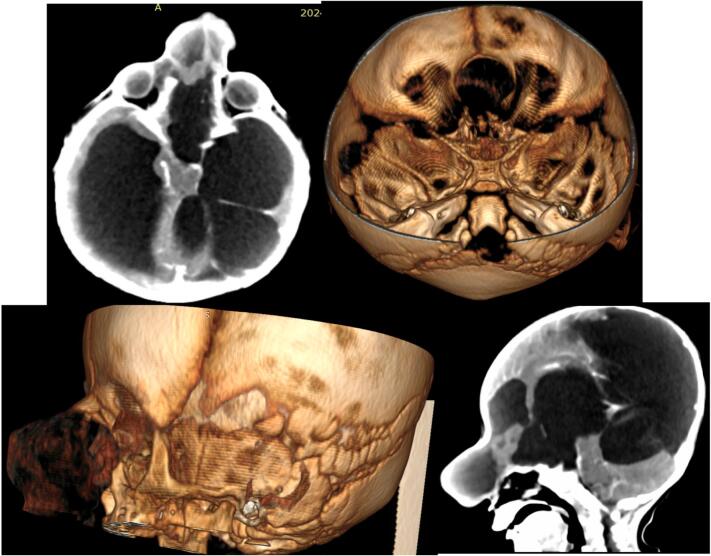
CT scan with 3D reconstruction showing the bone defect, protrusion of intracranial contents and hydrocephalus.
